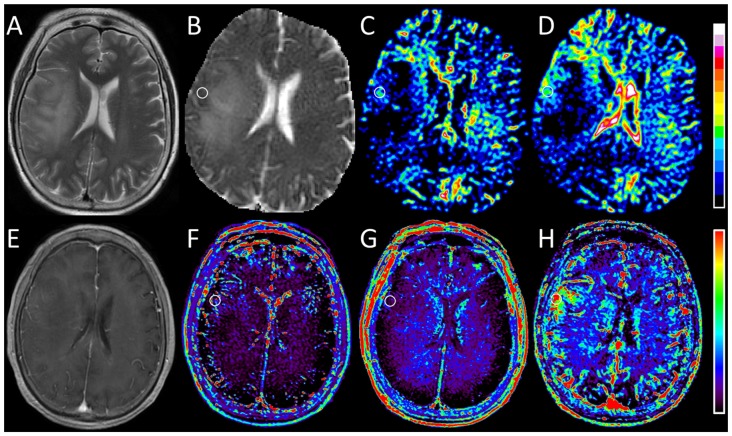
Images obtained in a 67-year-old woman with oligodendroglioma (WHO grade II). (A) T2-weighted image shows a hyperintense lesion with massive edema in the right hemisphere. (B) ADC_0,1000_ map shows increased apparent diffusion coefficient (ADC) value in the edema and slightly decreased ADC value in the corresponding area of the contrast-enhanced lesion as shown in (E). (C,D) IVIM *f* and SPF maps show relatively increased *f* and SPF values in the corresponding low-diffusion tumoral area compared with surrounding edema. (E) Contrast-enhanced T1-weighted image shows faint enhancement in the tumor. (F,G) DCE MR imaging parametric maps of K^trans^ and v_e_ show almost isointensity in the corresponding area of the contrast-enhanced lesion. (H) DCE MR imaging parametric map of v_p_ shows increased v_p_ value in the corresponding area of the contrast-enhanced lesion. Note that the tumoral hyperperfusion area on v_p_ map is consistent with the region on *f* and SPF maps. Round-shaped regions of interest are marked on parametric maps.
